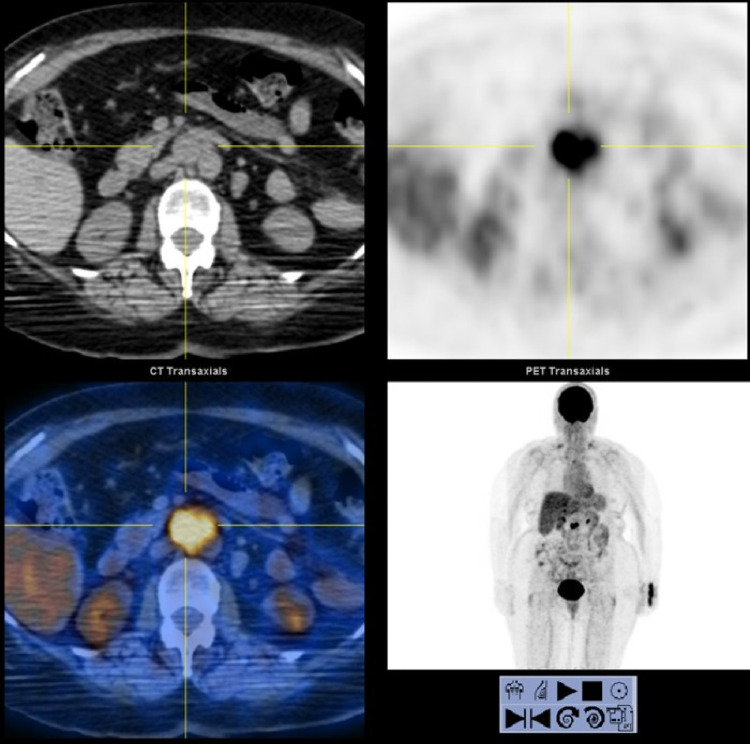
Positron emission tomography (PET) scan from case 3 showing para-aortic lymphadenopathy, with standard uptake value (SUV) 18.5
